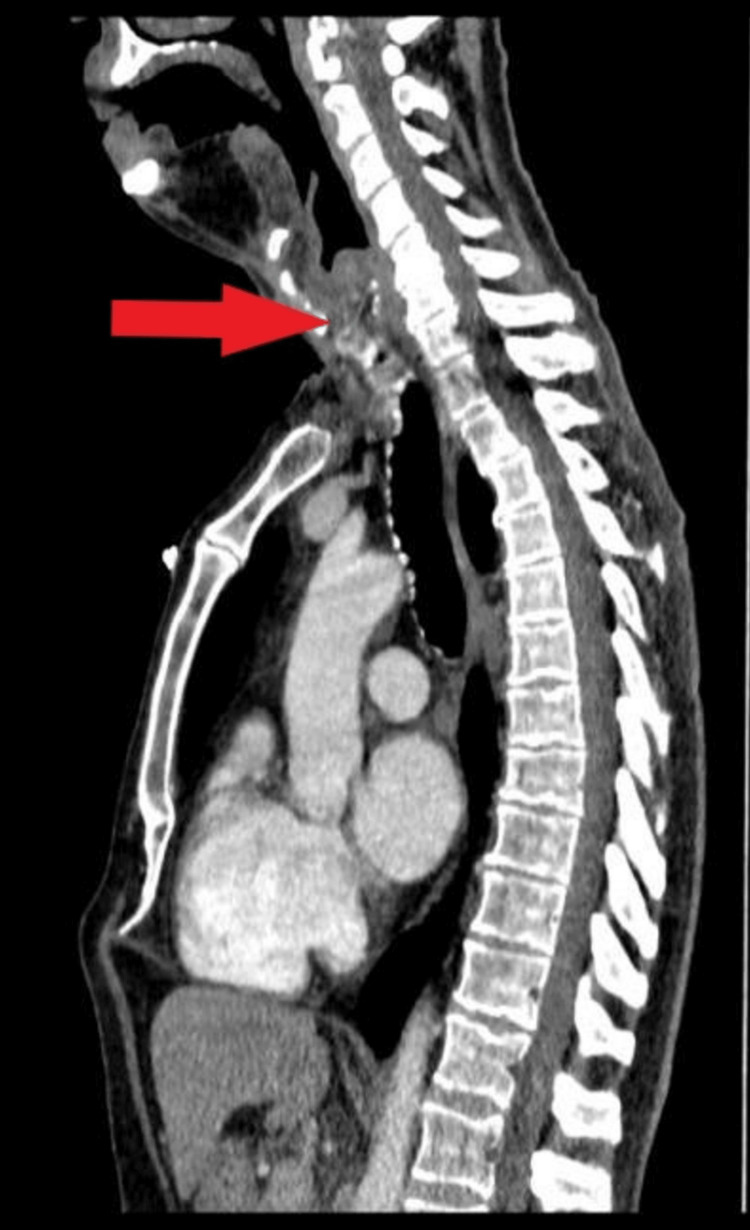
CT scan: red arrow pointing towards subglottic stenosis.
